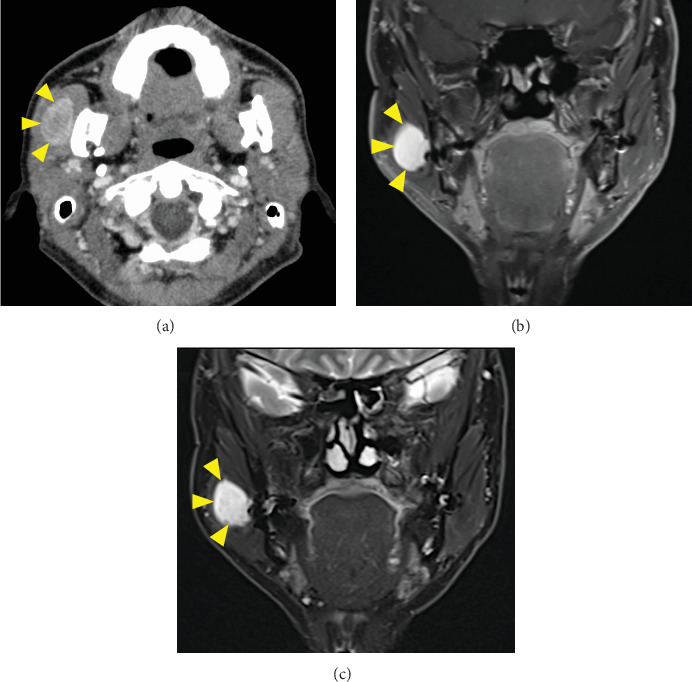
CT and MR images. (a) CT shows a well-defined 33 × 22-mm mass in the right masseter muscle showing low density in the center and slightly higher density at the margins of the mass. (b) T1-weighted and (c) T2-weighted MR images show a high-intensity mass in the right masseter muscle.
